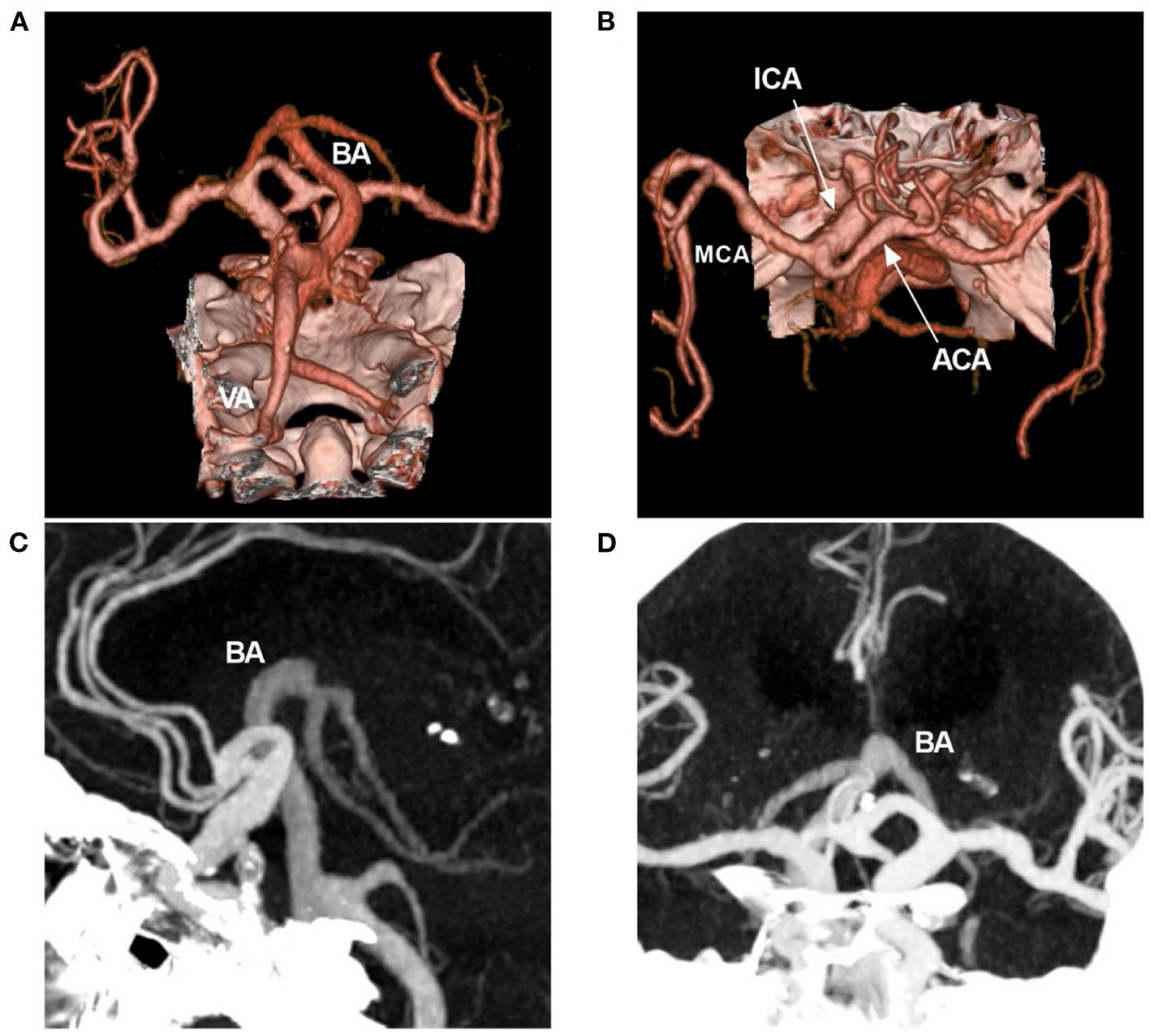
Diffuse intracranial dolichoectasia on CTA. (A,B) CTA reconstruction images showing diffuse intracranial dolichoectasia involving the anterior and posterior circulations. (C,D) MIP images showing that the BA tip reached the level of the lateral ventricle, the hydrocephalus was found due to the VBD compression. ACA, anterior cerebra artery; BA, basilar artery; CTA, computed tomography angiography; ICA, internal carotid artery; MCA, middle cerebral artery; MIP, maximum intensity projection; VA, vertebral artery; VBD, vertebrobasilar dolichoectasia.
